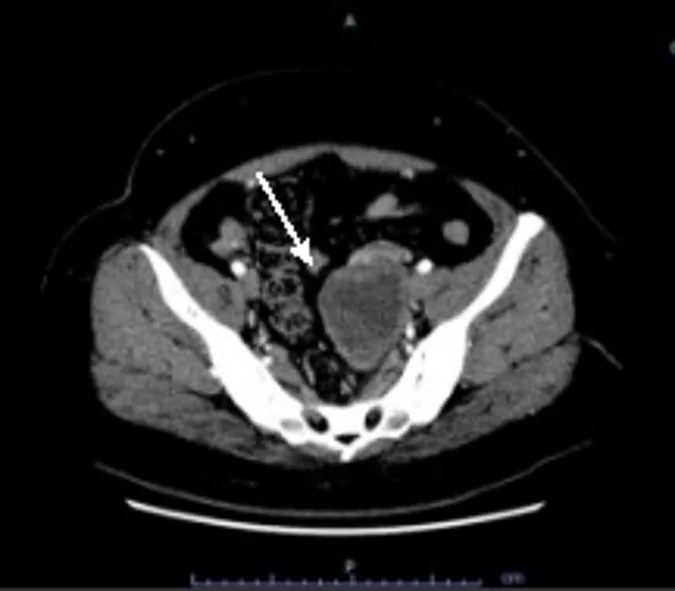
Contrast-enhanced CT shows a 7.5 cm multilocular cystic lesion in the left ovary, characterized by poor contrast enhancement, and suggesting blockage of blood flow leading to ischemia and necrosis of ovarian tissue (arrow)
